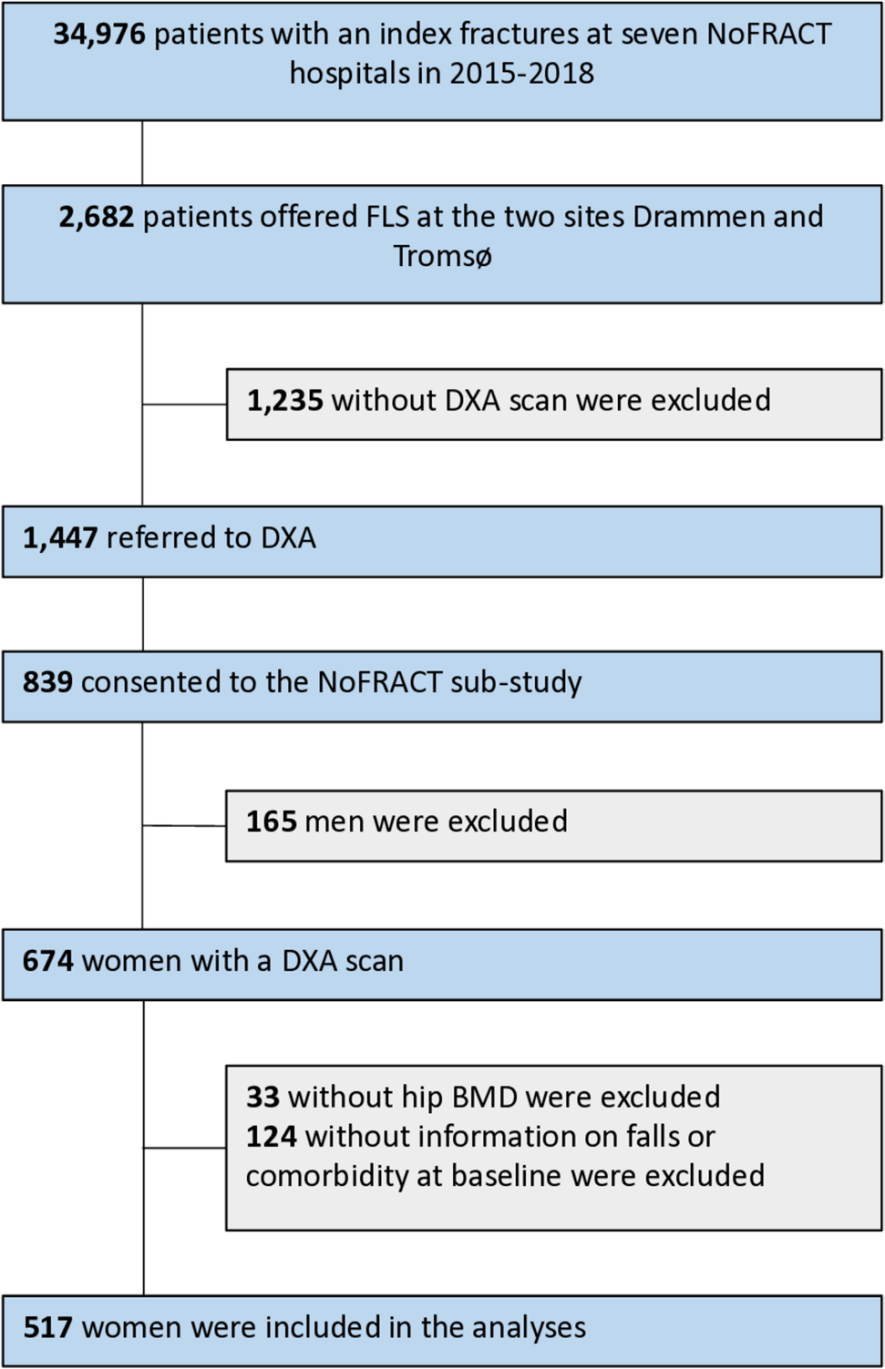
Flow-chart of the study participants. NoFRACT, Norwegian Capture the Fracture Initiative study; DXA, dual energy X-ray absorptiometry; FRAX, Fracture Risk Assessment Tool; BMD, bone mineral density
